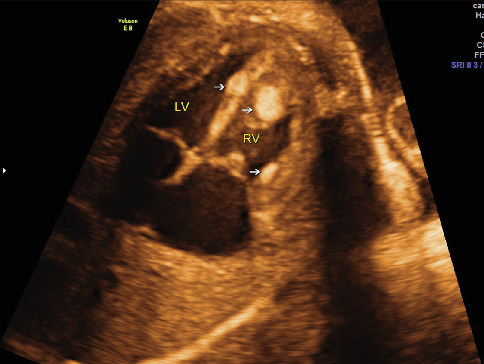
Fetal echocardiography showed multiple masses in the right ventricular and ventricular septum (as indicated by the arrow).
